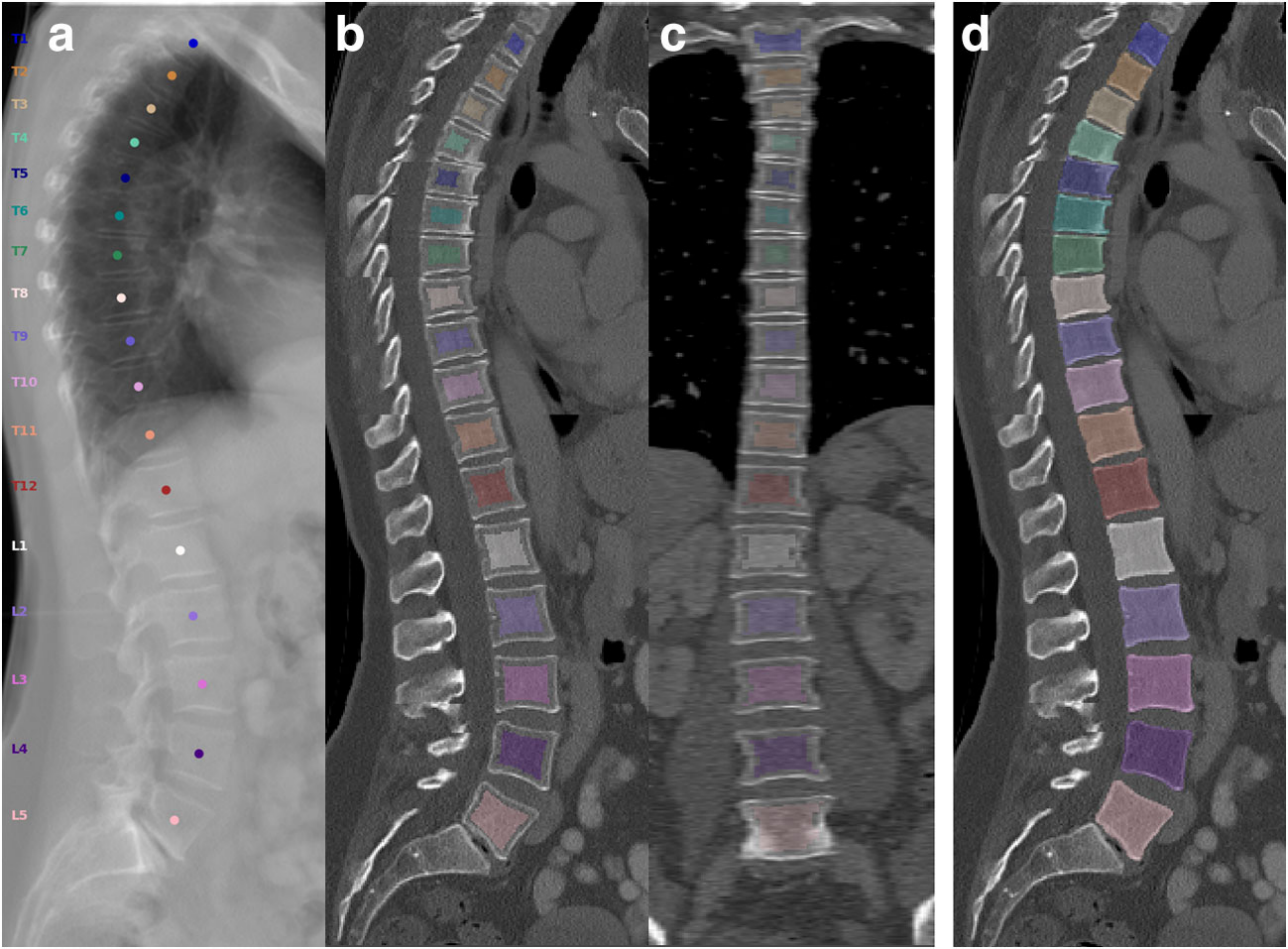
CT scan of a 50-year-old woman performed for breast cancer staging visualized as virtual radiograph in lateral projection (a) and curved planar reconstructions in lateral and coronal views (b, c, d). No osteoporotic vertebral fracture is visualized. There are signs of spondylosis at L5/S1. Assessment of T12 to L4 yielded a mean trabecular vBMD of 134.7 mg/cm^3^, integral vBMD of 204.5 mg/cm^3^, CT-based aBMD of 1.008 g/cm^2^, and BMC of 6.18 g. DXA reported T-score of − 2.2 SD (not shown). Trabecular and integral vBMD concur with normal bone mass. DXA T-score corresponds to low bone mass with tendency towards the threshold for osteoporosis (< − 2.5 SD). Masks for extraction of trabecular (b, c) as well as integral vBMD (d), are shown as colored overlays. For more details on image creation please refer to Fig. 2 and “Methods”
